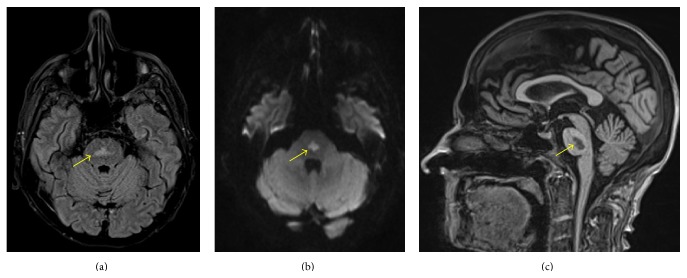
Brain magnetic resonance imaging shows a lesion in the central pons with imaging characteristics consistent with demyelination. The lesion is hyperintense on both (a) Fluid-Attenuated Inversion Recovery Image and (b) Diffusion-Weighted Imaging. The lesion is hypointense on (c) T1-weighted sequence.
